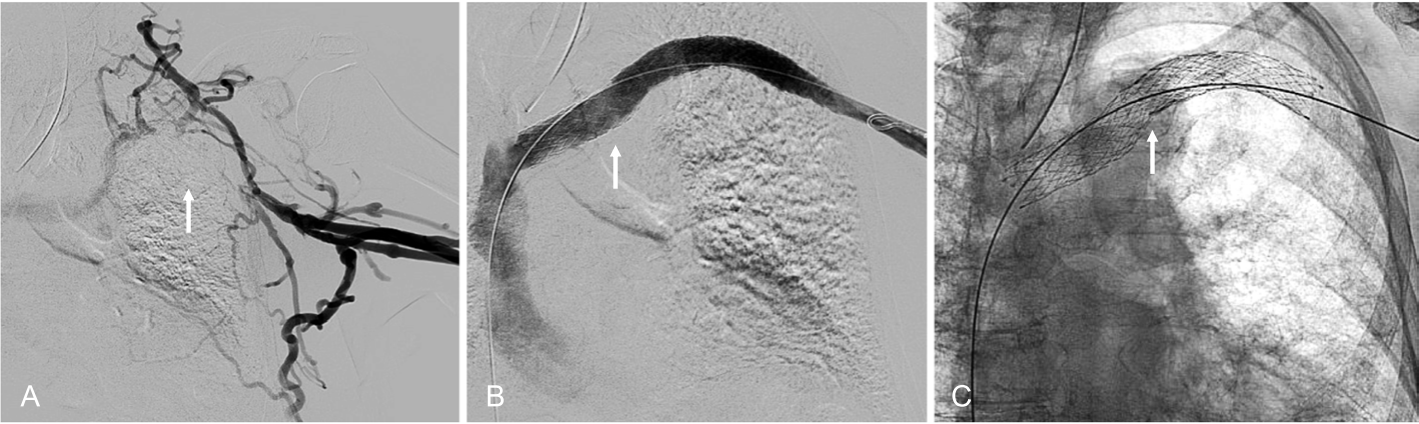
76-year-old man with recurrent left axillosubclavian venous thrombosis and left upper extremity swellin*g (A) Left upper extremity venography demonstrating left axillosubclavian venous occlusion (arrow) (B) Left upper extremity venography, after left axillosubclavian stent reconstruction using 12-mm and 14-mm Abre Venous Stents (arrow), showing brisk in-line from the left upper extremities to the right atrium (C) Radiograph demonstrating the 12-mm and 14-mm Abre stent in the thoracic outlet (arrow) without deformation or fracture
